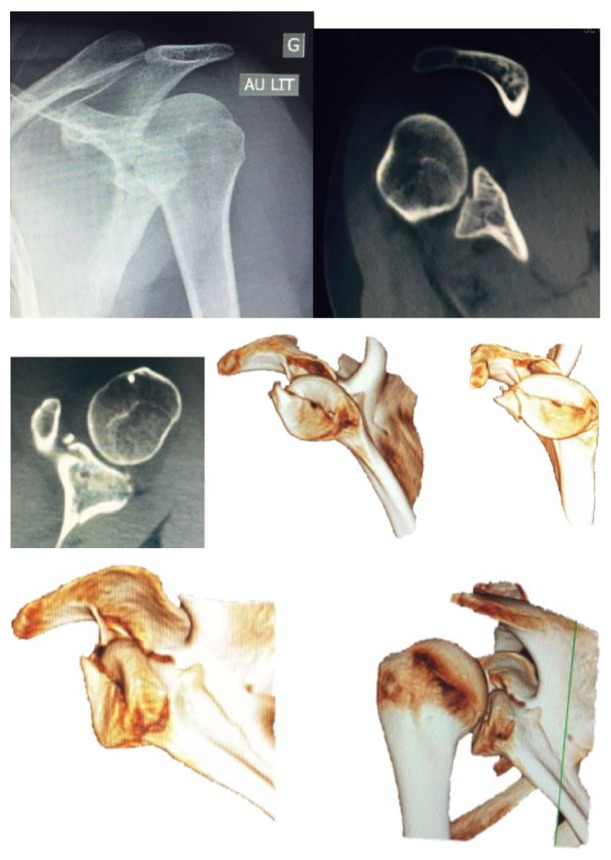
X-ray and CT with 3D images of an articular glenoid fracture.
